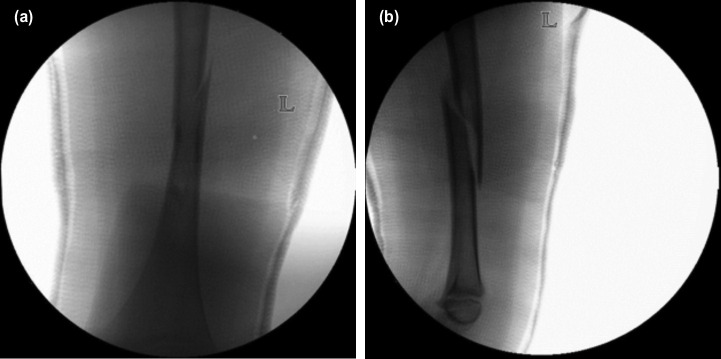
(a,b) Radiographs of the closed reduction and hip spica application of patient A.
